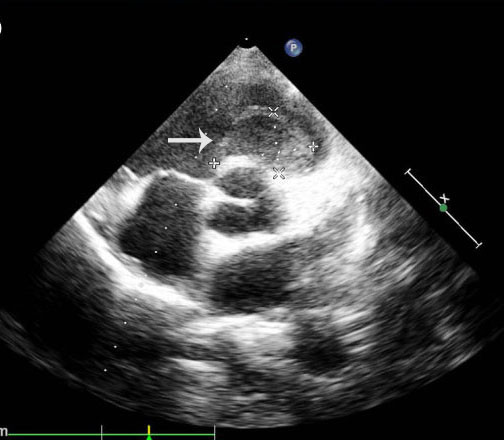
Preoperative, transthoracic echocardiographic scan (parasternal short-axis view of aortic root). A tumor measuring approximately 5cm × 5cm and extending from the right ventricle to the pulmonary valve can be seen (arrow).
